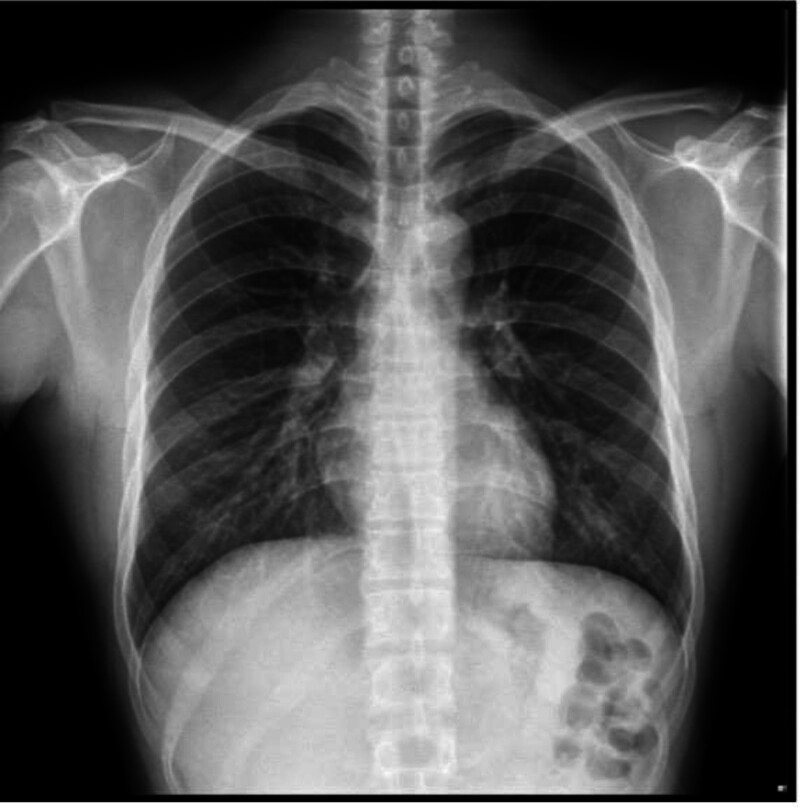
Preoperative chest radiograph (May 15, 2025): normal bilateral lung fields with preserved costophrenic angles and absence of consolidation.
